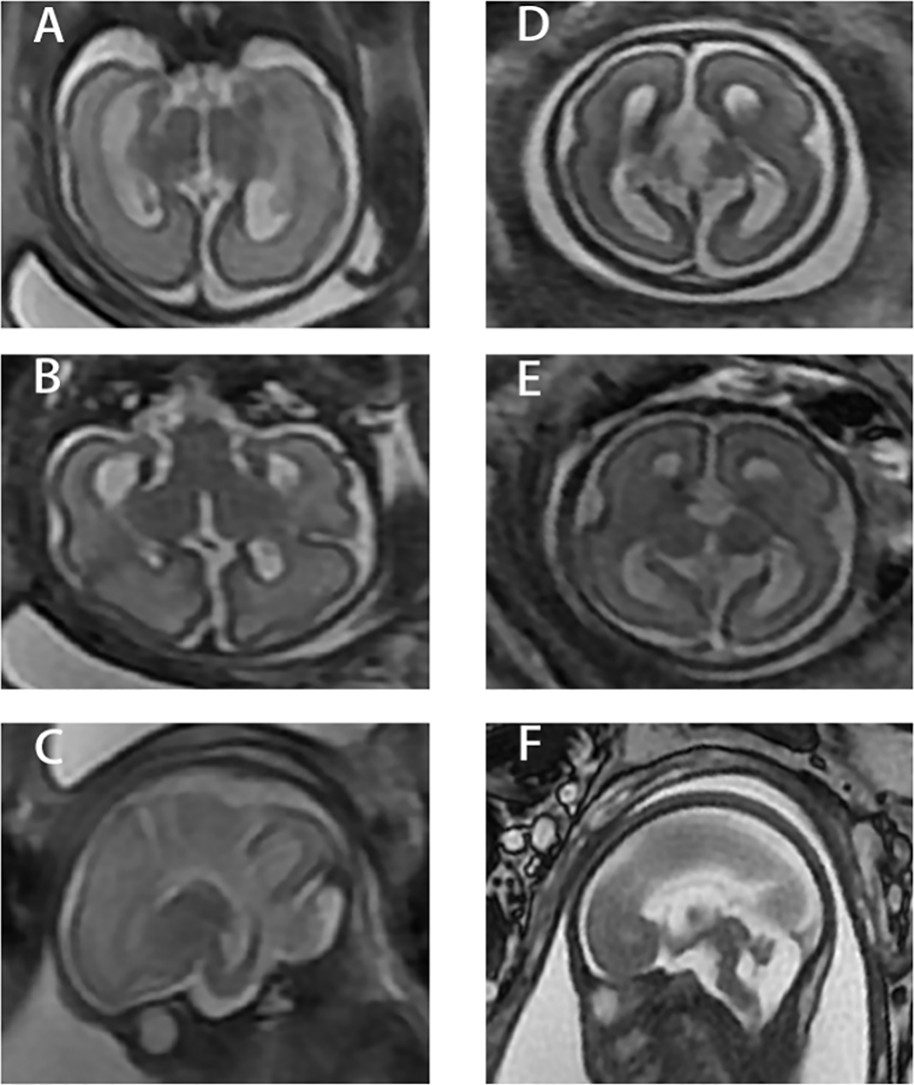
Three different sections of the two cases. (A–C) Case 1. (D–F) Case 2. 1) (A) and (D) are coronal images from fetal MRI. 2) (B) and (E) are axial images from fetal MRI. 3) (C) and (F) are sagittal images from fetal MRI.
